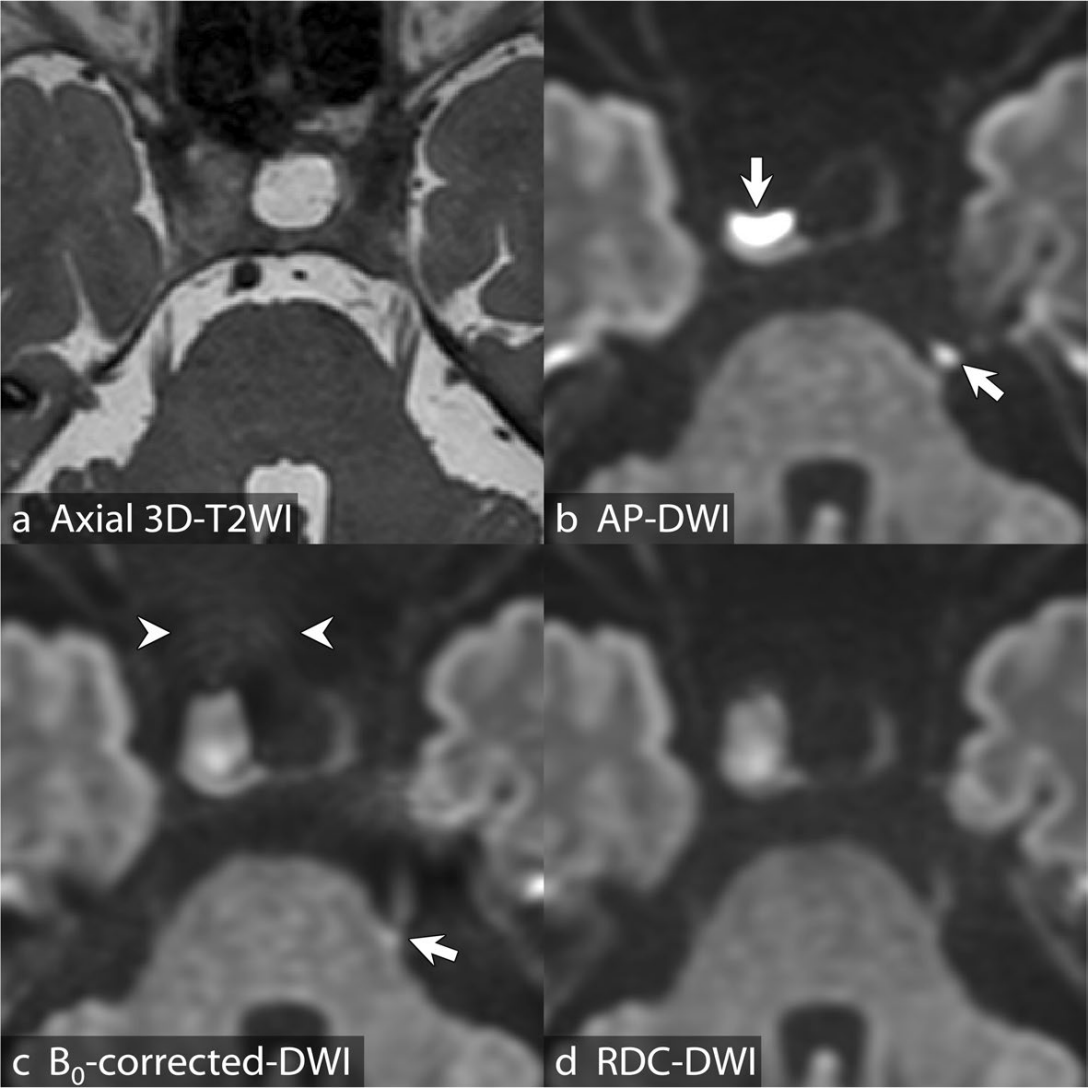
A 68-year-old woman with residual non-functioning PitNET/pituitary adenoma after surgery. Axial 3D-T2WI (a), axial *b* = 1,000 s/mm^2^ images of AP-DWI (b), B_0_-corrected-DWI (c), and RDC-DWI (d) are shown. The residual PitNET/pituitary adenoma cannot be clearly distinguished from the right cavernous sinus on axial 3D-T2WI (a). There is severe distortion, susceptibility artifact, and signal pileup around the residual PitNET/pituitary adenoma and the left trigeminal nerve on AP-DWI (b, arrows). B_0_-corrected-DWI and RDC-DWI depict undistorted residual PitNET/pituitary adenoma without apparent susceptibility artifact (c and d). Compared with B_0_-corrected DWI, there is less blurring on RDC-DWI, particularly in the sphenoid sinus (c, arrowheads), and there are fewer susceptibility artifacts in the left trigeminal nerve (c, arrow). *3D-T2WI*, Three-dimensional T2-weighted imaging; *AP*, Anterior-posterior; *DWI*, Diffusion-weighted imaging; *PitNET*, Pituitary neuroendocrine tumor; *RDC-DWI*, Reverse encoding distortion correction DWI
